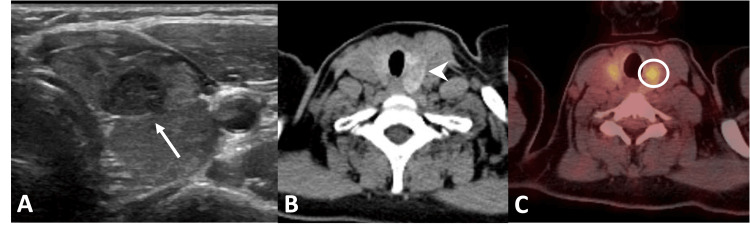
Breast cancer metastasis to the thyroid gland. (A) Gray-scale ultrasound of the left thyroid lobe demonstrates a well-circumscribed, heterogeneous, hypoechoic solid nodule (white arrow). (B) Axial unenhanced CT shows a heterogeneous hypodense nodule (arrowhead) in the left thyroid lobe. (C) Axial 18F-FDG PET-CT demonstrates mild FDG uptake within the nodule (circle), consistent with metastatic involvement. ^18^F-FDG PET-CT: Fluorine-18 fluorodeoxyglucose positron emission tomography/computed tomography; CT: Computed tomography; FDG: Fluorodeoxyglucose
